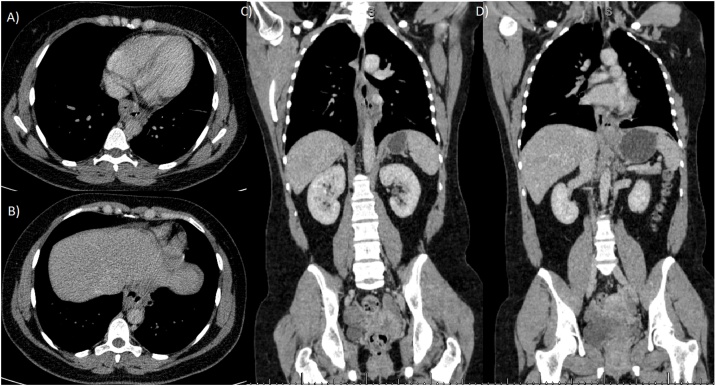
Computed tomography. A and B: axial view, showing a confined collection with air in the lower mediastinum surrounding esophagus. C and D: coronal view showing a confined periesophageal collection, and distal esophageal wall thickening.
